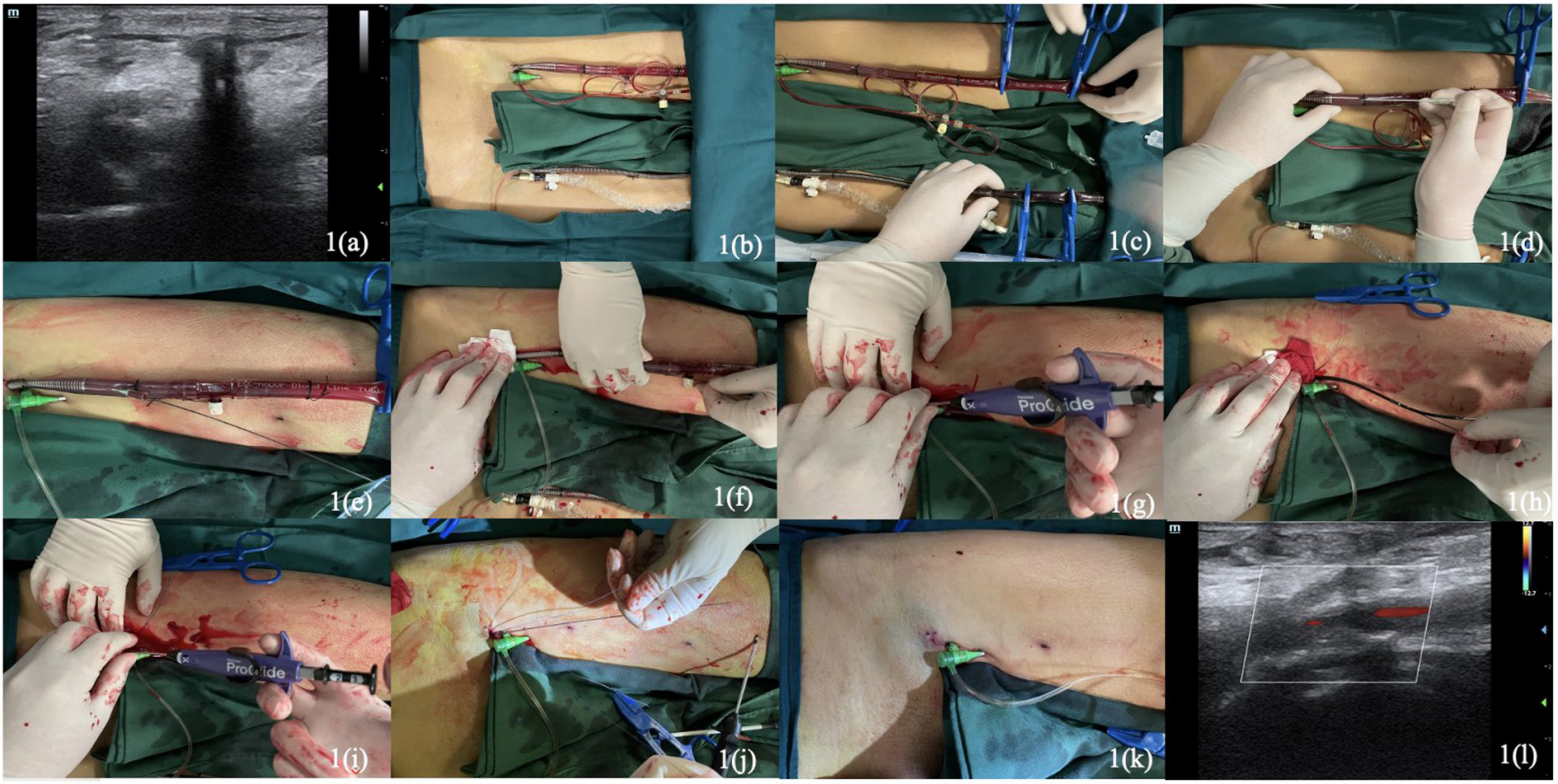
Pre-operative ultrasound images (a). Sterile preparation of the operative area (b). The clamping of arterial and venous cannulas at the distal part by four tubing clamp forceps (c). Direct puncture with seldinger technique in the proximal portion of the arterial cannula (d). The insertion of Terumo Stiff guidewire through the cannula (e). Removal of the arterial cannula under manual compression at the access by an assistant (f). Insertion of the first Perclose Proglide and deploying at the direction about 10–11 o' clock (g). Removal of the first Proglide and placement a clamp forcep to hold the two suture limbs together on the right side of the patient (h). Reinsertion of the guidewire to place the second Proglide and deploy at about 1–2 o' clock (i). Wrapping the railed suture limbs tightly around the left index finger to pull it (j). The hemostasis was adequate and cut the suture tails (k). Post-operative ultrasound images with normal blood flow of femoral artery (l).
